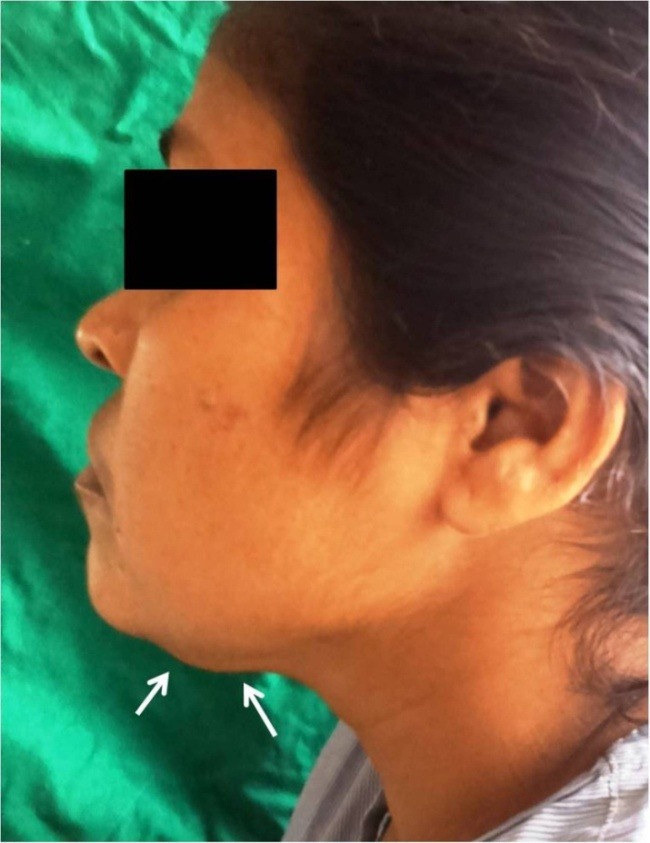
An orthopantomogram showed a well-defined homogenous radiopaque mass arising from basal part of the mandible below the apices of the left premolar and the first molar teeth with a continuous contour (Figure 2). Computed tomography (CT) scan with sectional and 3D formatted images was obtained for further evaluation of the lesion. CT scan showed a well-defined, hyperdense mass measuring 2.5×2 cm in size, arising from the left lower border of the mandible. Coronal and sagittal sections showed the growth projecting slightly towards the lingual aspect and impinging over the muscle plane on the oral cavity floor and neck (Figure 3). No other lesion was seen involving the craniofacial skeleton. Based on the clinical and radiological findings, provisional diagnosis of peripheral osteoma was made.
